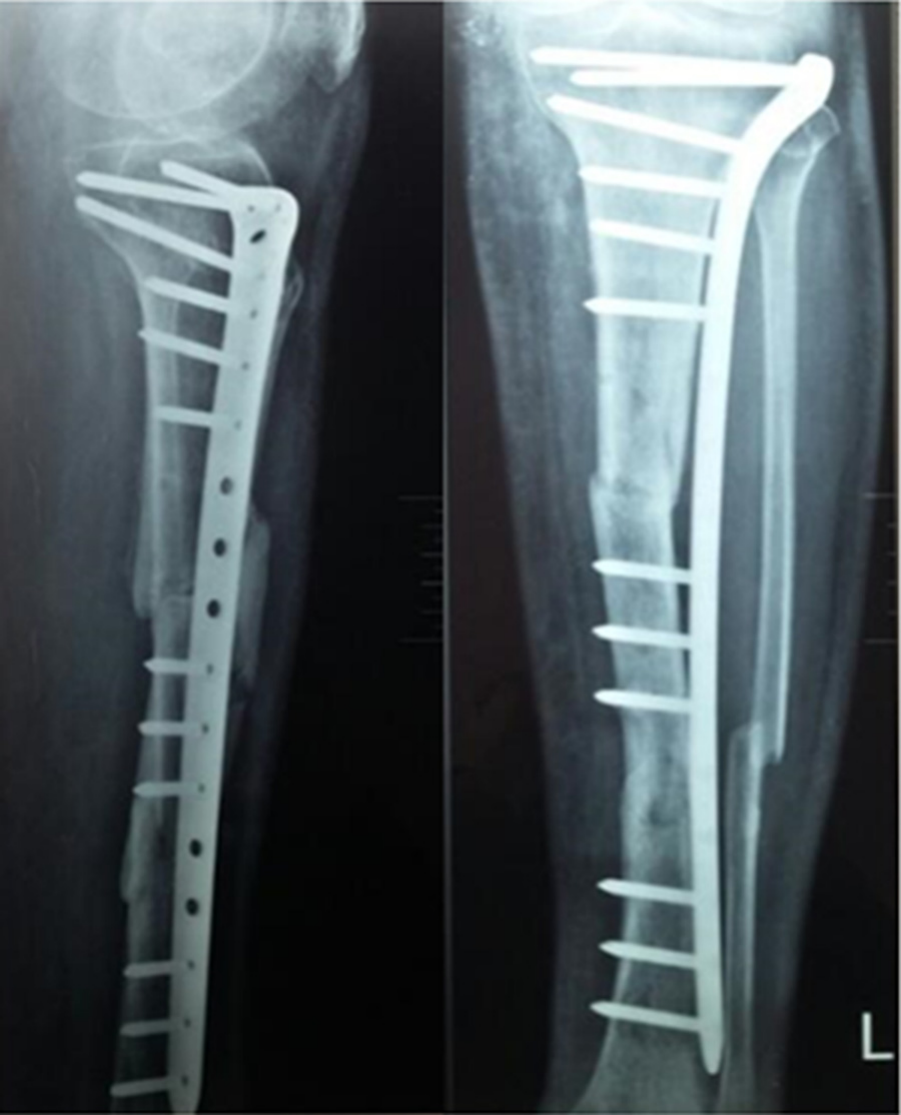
AP and Lat radiographs showing healed proximal and distal fractures 20 weeks postoperatively.
